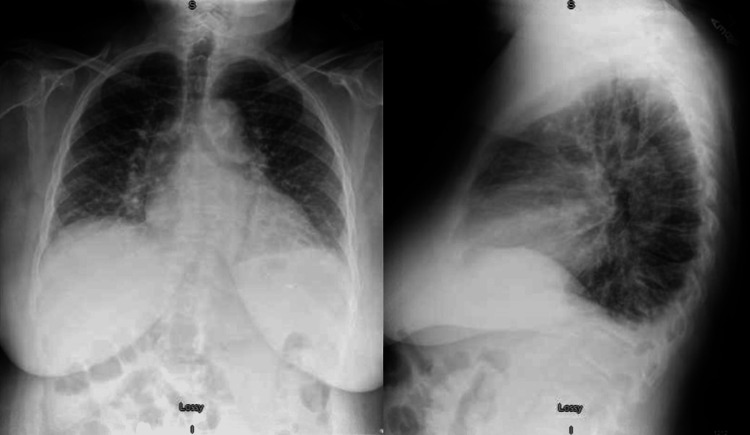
Chest X-ray on admission demonstrated pulmonary edema and borderline cardiomegaly, but without pleural effusion
